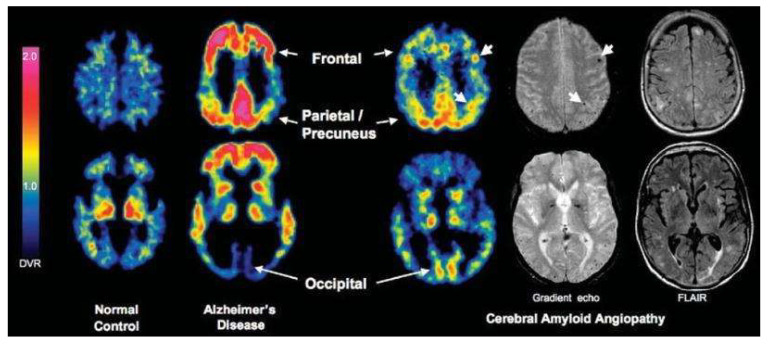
Multimodal imaging biomarkers relevant to ARIA risk prediction. PET demonstrates amyloid burden in Alzheimer’s disease, while GRE and FLAIR MRI show microhemorrhages and white matter hyperintensities. When combined with APOE ε4 status and treatment factors, these imaging markers contribute to ARIA risk stratification. Created in BioRender. Christodoulou, R. (2025) https://BioRender.com/xcro709 (accessed on 2 September 2025).
